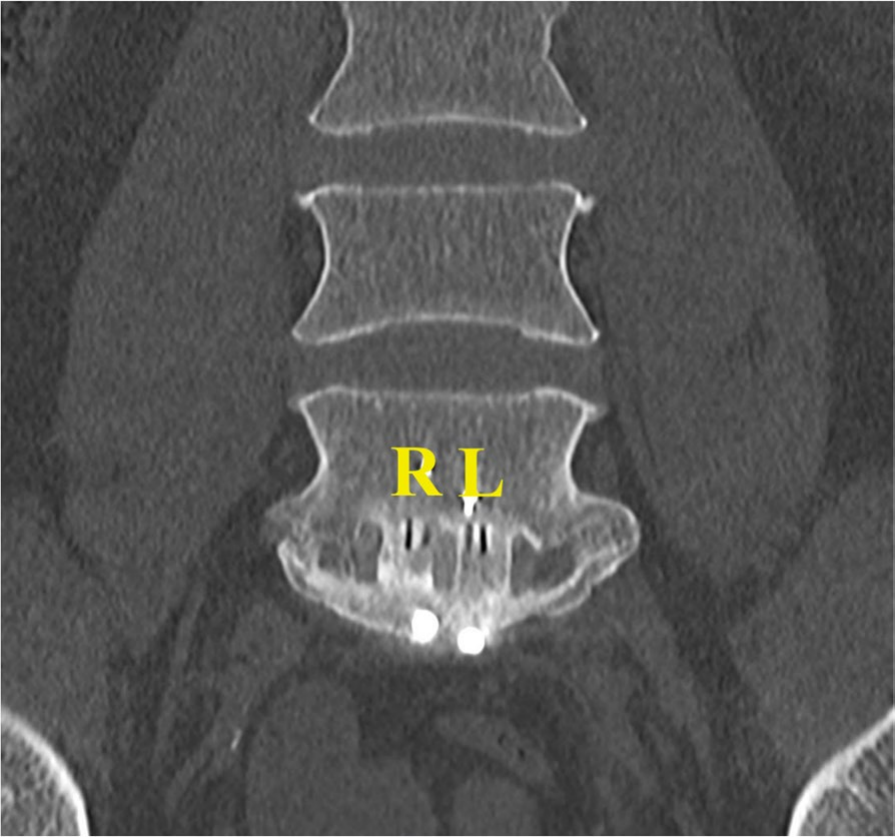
Frontal CT scan of a patient with fusion of Bridwell grade I1 in both the chamber filled with bioactive glass (R) and the chamber filled with autologous bone (L)
